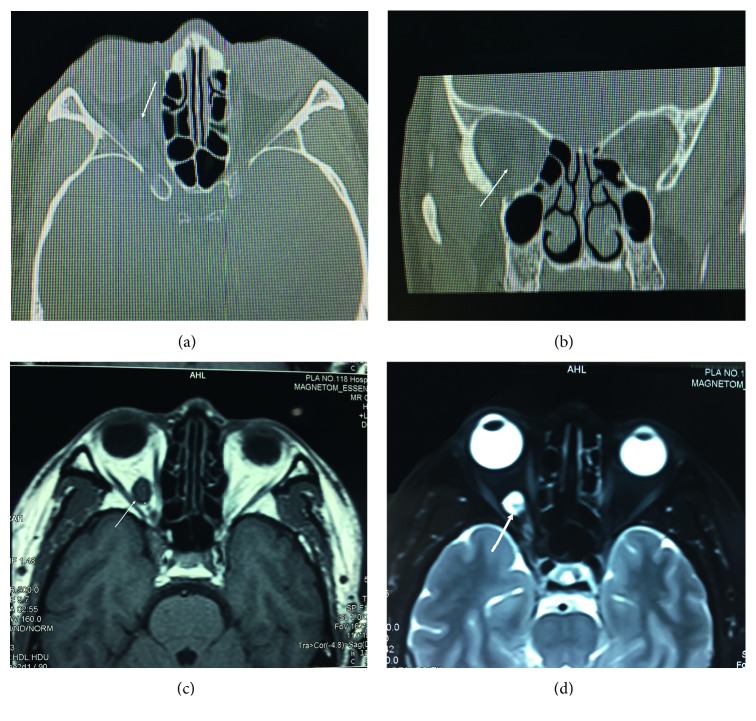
Computed tomography (CT) and magnetic resonance imaging (MRI) of the patient with schwannoma at the apex. (a, b) Orbital CT images showed a suborbicular and well-defined mass with isodensity mass located at the right inferolateral apex. (c) MRI T1-weighted images showed an isointensity mass at the right apex. (d) MRI T2-weighted images showed a high-intensity mass (white arrows).
